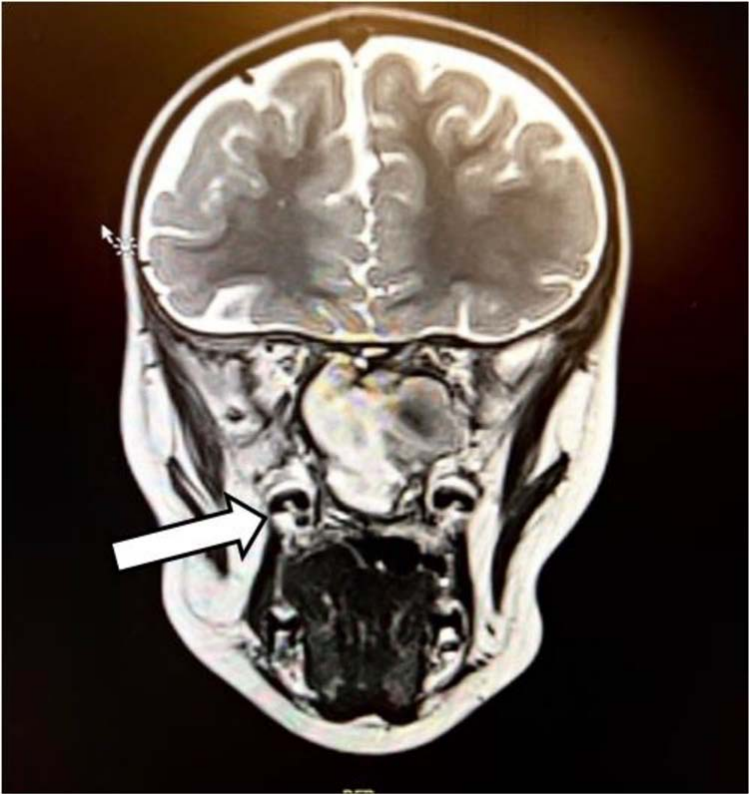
The coronal section of the MRI.
